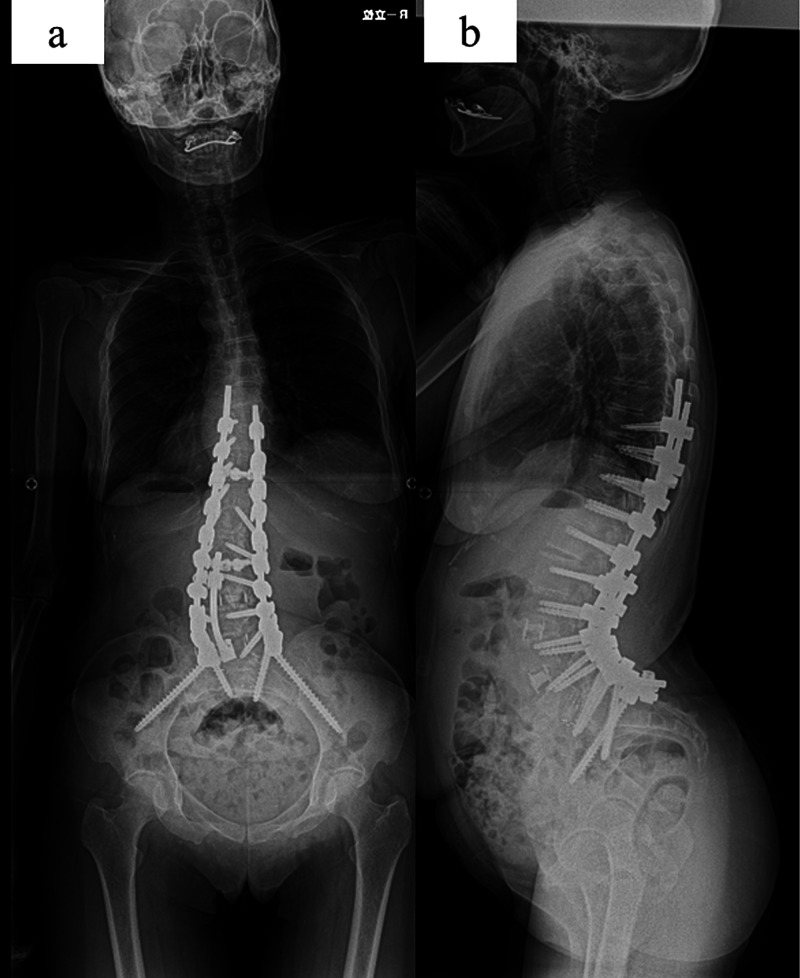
Postoperative images Postoperative posterolateral (a) and lateral (b) standing radiographs. The patient underwent combined anterior (L3/4, L4/5) extreme lateral interbody fusion and posterior fixation (T9-ilium), restoring significant lumbar lordosis and improving global coronal and sagittal balance.
